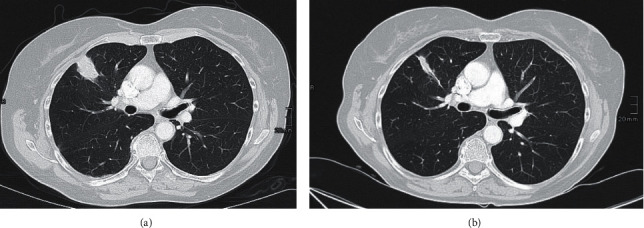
Chest computed tomography scans. (a) The initial scan shows a solid nodular lesion with irregular margins and adjacent to the visceral pleura in the right lower part of the upper lobe. (b) Five years later, the CT scan revealed only a small scar in the former lesion.
